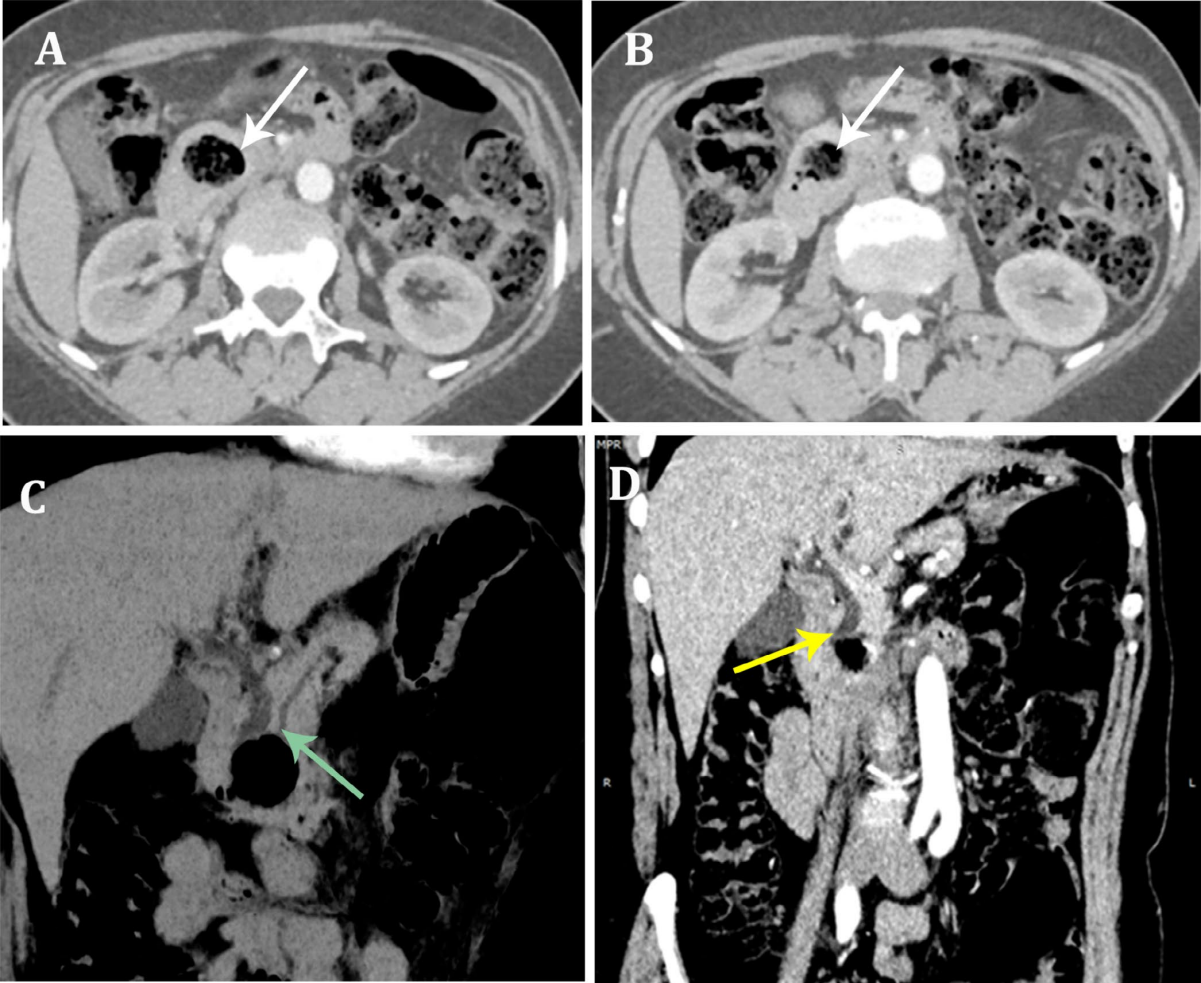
(A and B) Axial CT images showing duodenal diverticulum (white arrows). (C and D) Oblique coronal CT images with non‐dilated main pancreatic duct (green arrow) and external compression of common bile duct by the diverticulum (yellow arrow).
